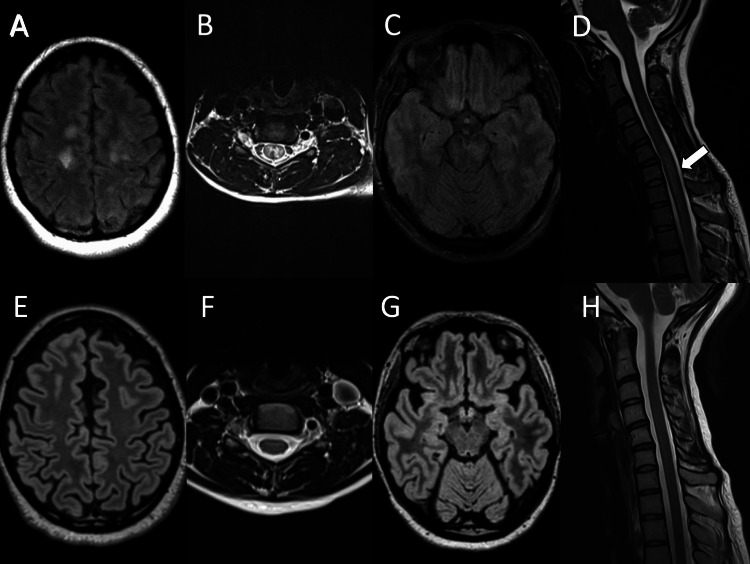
MRI brain and cervical spine from Case 1 at initial presentation. FLAIR MRI, axial view, showing subcortical frontal hyperintense foci (A); T2 MRI, axial view, of cervical spine showing hyperintense grey matter, H-sign (B); FLAIR MRI, axial view, showing left midbrain involvement (C); and T2 MRI, sagittal view, of cervical spine, arrow showing hyperintense cord signal (D). Interval resolution of T2-hyperintense lesions on subcortical frontal lobes (E), cervical spine (F), midbrain (G), and cervical spine on follow-up imaging three months later (H). MRI: magnetic resonance imaging; FLAIR: fluid-attenuated inversion recovery image
